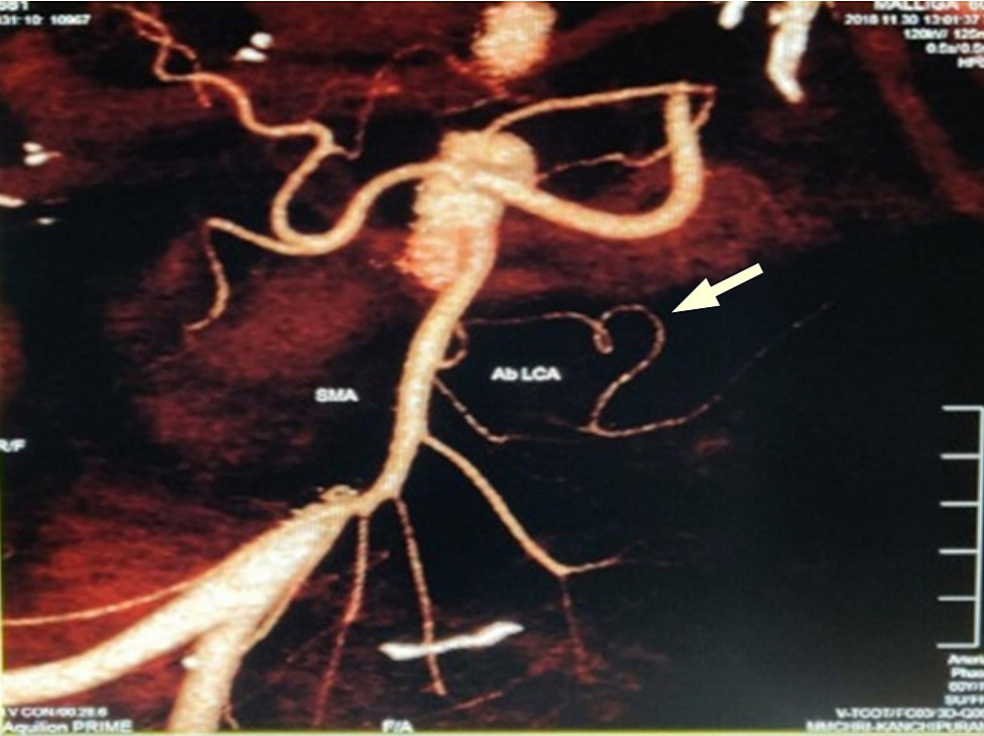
CT angiogram denoting ab LCA. CT angiogram of abdomen denoting ab LCA with an arrow pointing towards it ab LCA, aberrant left colic artery
